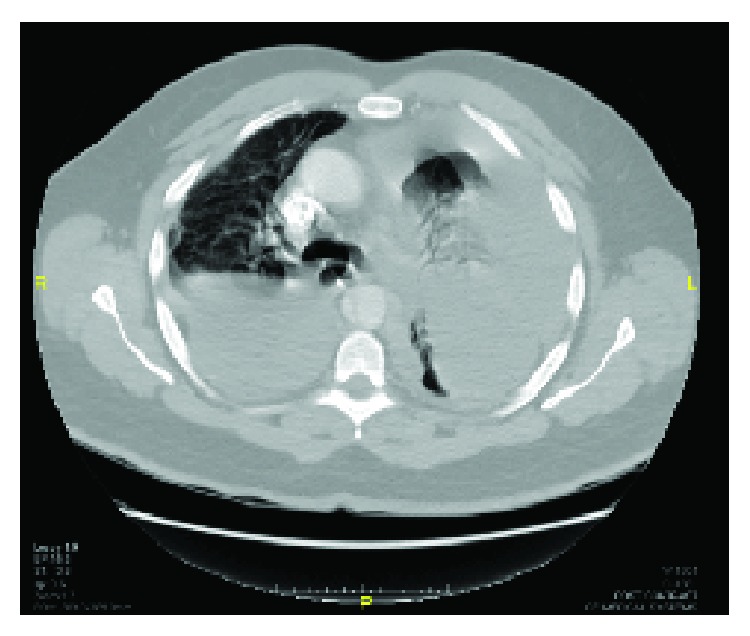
Another image of the CT chest (axial) in the lower chest showing cuts with massive hemothorax.
